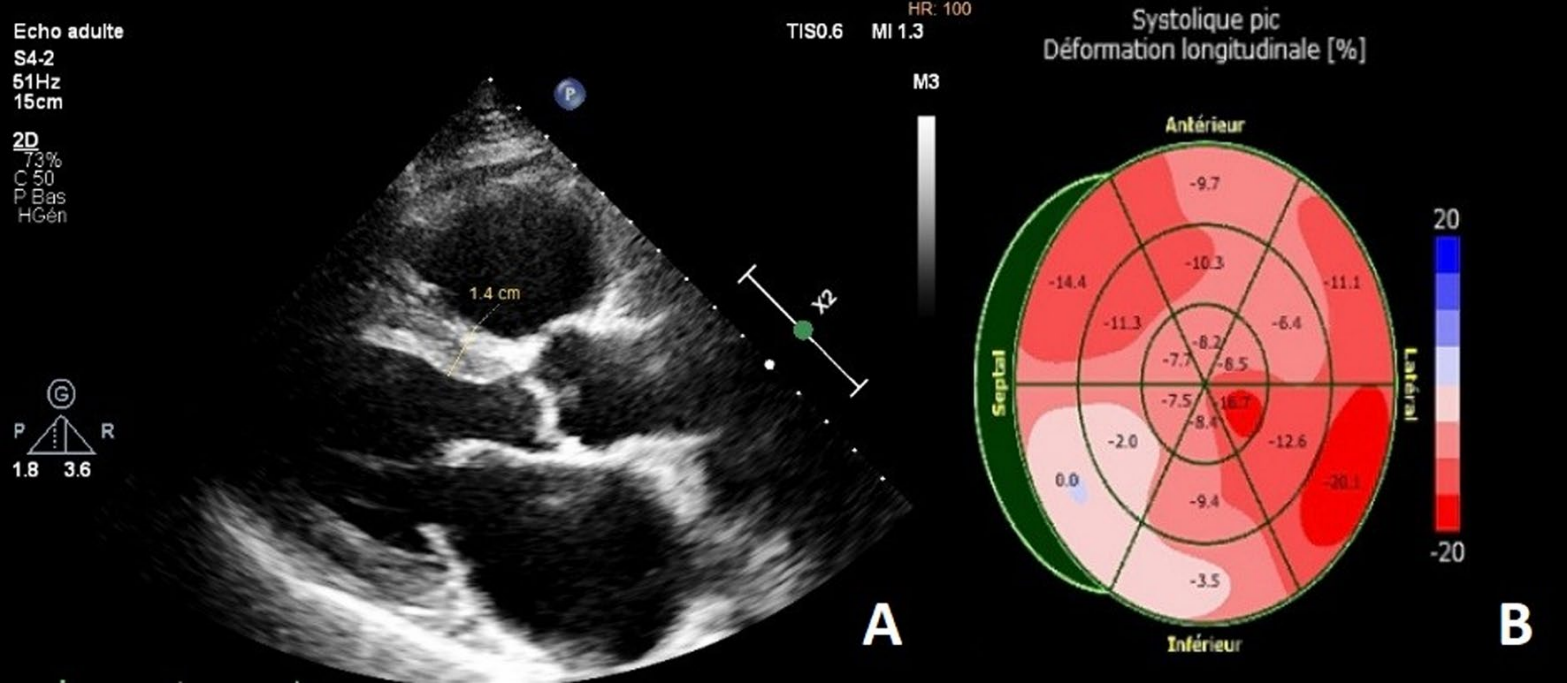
Transthoracic echocardiography revealing concentric hypertrophy with mildly reduced LVEF (43%). A The parasternal long-axis view shows an enlarged left atrium, normal valves structure with no calcification, septal and posterior wall hypertrophy. B The bull’s-eye plot from two-dimensional speckle-tracking echography visualizing the regional wall motion abnormalities and reduced GLS 9.6%
